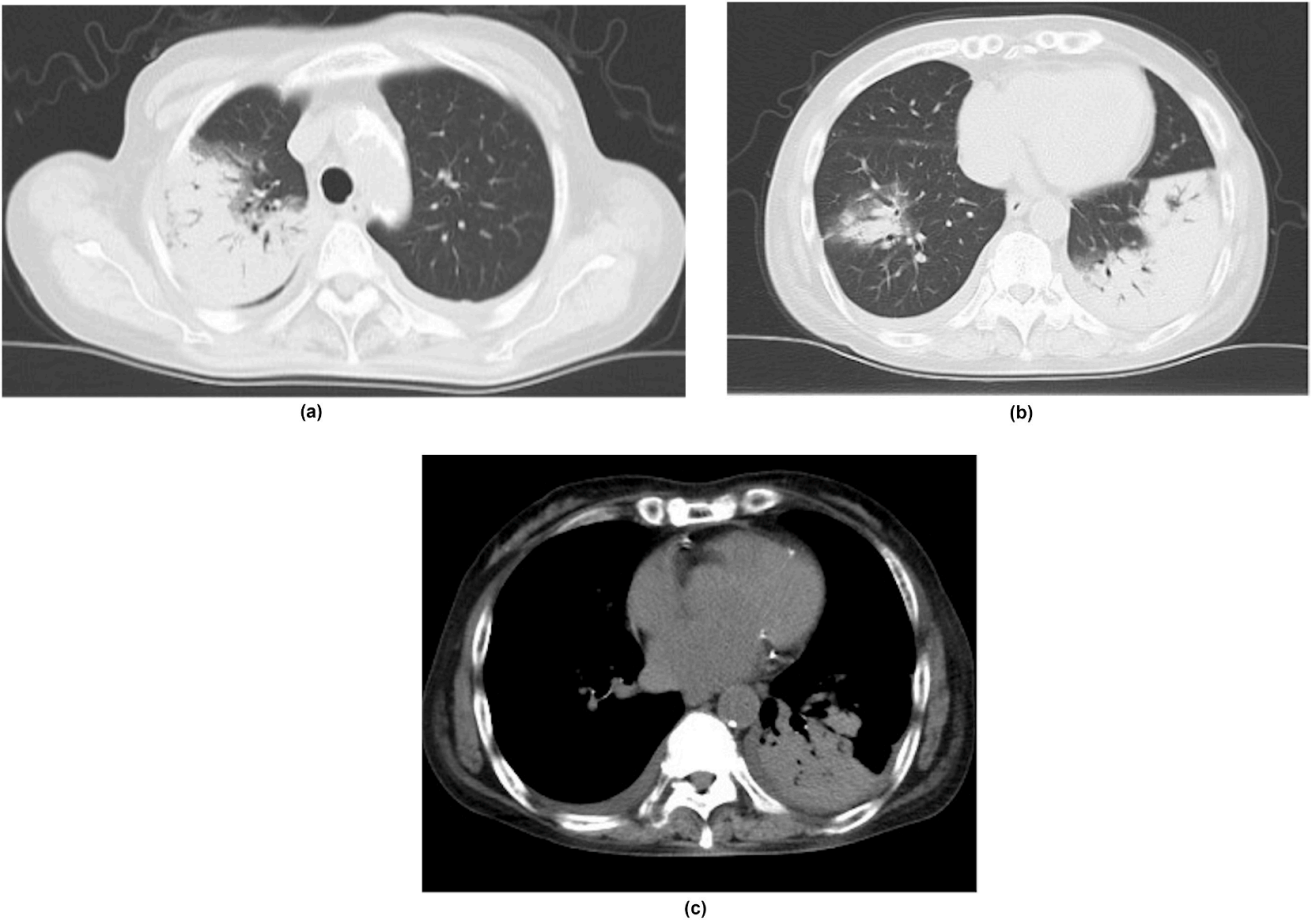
Chest CT at first examination. Chest CT showed multiple infiltrating shadows in the right upper and lower lobes and the left lower lobe. A small amount of pleural effusion was observed in both lung fields.
